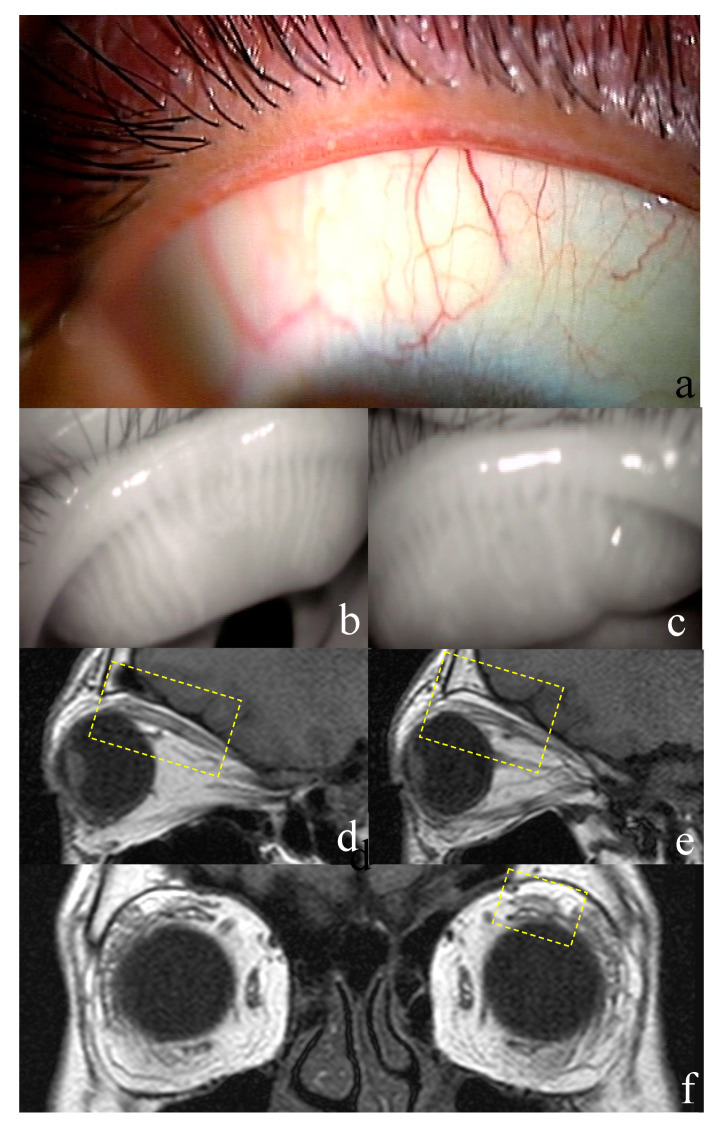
Representative case of a 53-year-old woman with Graves’ ophthalmopathy. The patient presented with vascular engorgement, lid-margin inflammation, and plugging of the lid margin (a). The meibomian glands at the central region of the eyelids exhibit abnormal findings, particularly in the upper eyelid (b,c). Magnetic resonance imaging findings show enlargement of the levator muscle and lacrimal gland (d–f).
